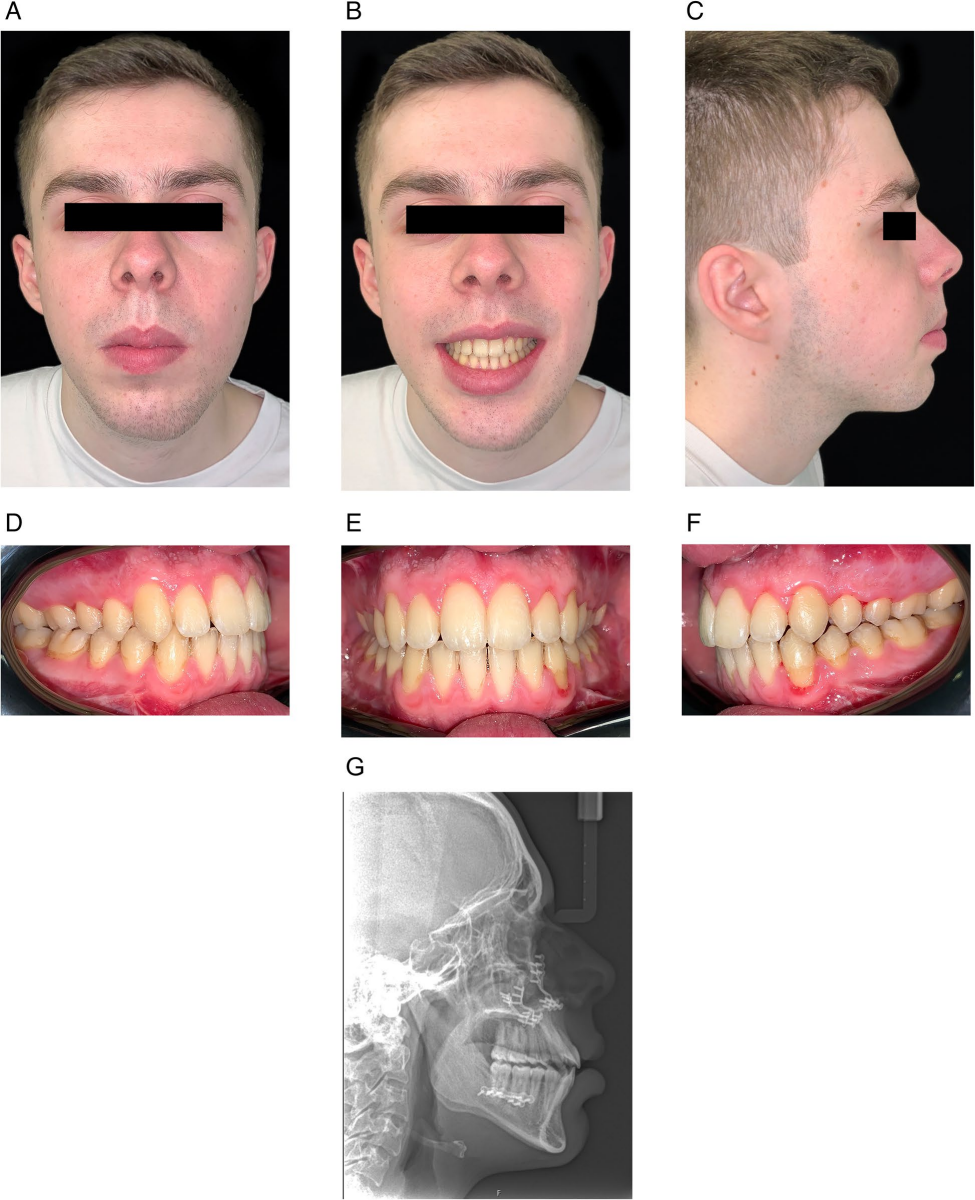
End-of-treatment clinical photographs and lateral cephalometric radiograph
